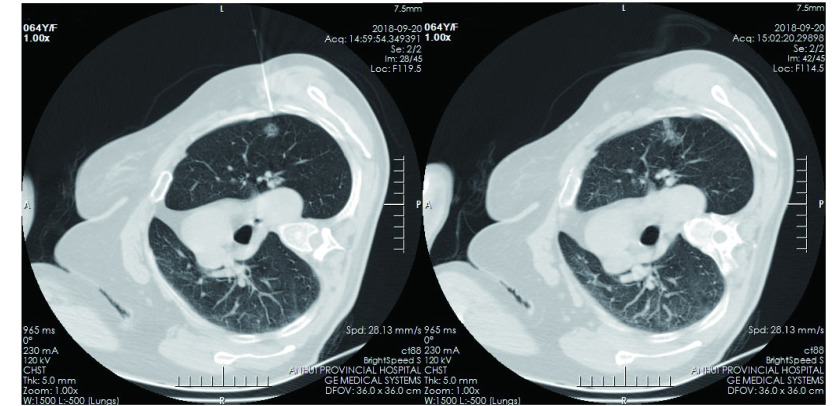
CT影像上形成靶区团块和注射针道组成的“蒲公英”效应 The "dandelion" effect is formed on CT image, which consists of target area mass and injection tract. CT: computed tomography.
